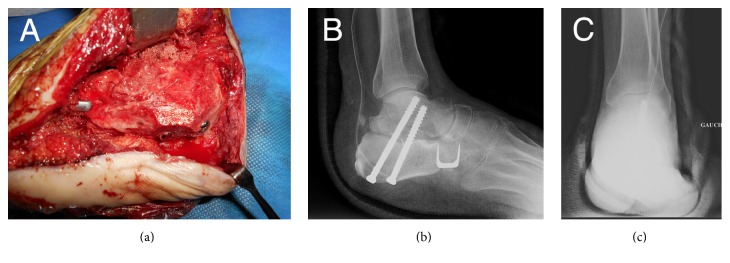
Intraoperative lateral view of the calcaneal allograft arthrodesis (a) and postoperative X-ray examination (b and c) of the calcaneal allograft and double arthrodesis.
